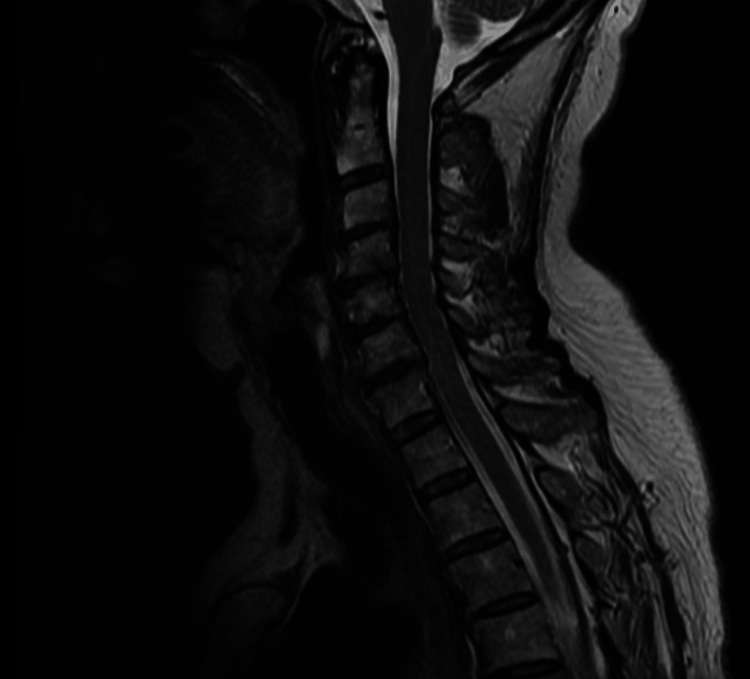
Sagittal view, T2-weighted MRI of the cervical spine, with no nerve root or leptomeningeal enhancement. Mild degenerative disc changes were present across C3-C7.
